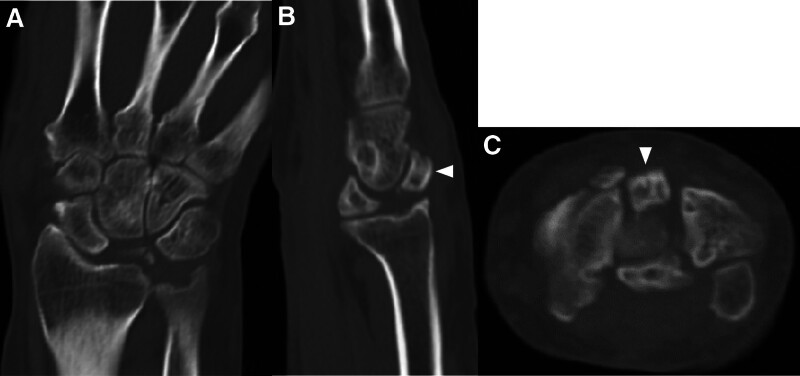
Initial plain computed tomography. (A) Coronal, (B) sagittal, and (C) axial view of right wrist showing displaced volar and dorsal segment of lunate bone (arrowhead).
